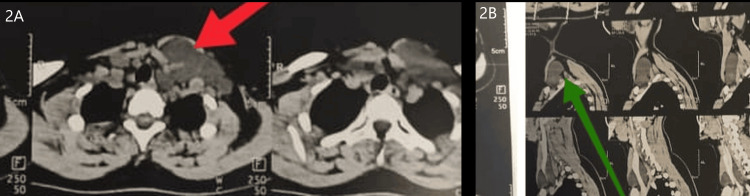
Contrast-enhanced cervicothoracic CT scan of a pediatric patient with cystic lymphangioma. (A) Axial images: the red arrow indicates a large multiloculated hypodense cystic lesion in the left lateral cervical region, well-circumscribed, with mild mass effect on adjacent cervical structures and no solid enhancing components. (B) Sagittal images: the green arrow demonstrates the cervicothoracic extension of the lesion, highlighting its craniocaudal spread across the cervical and superior mediastinal compartments. The overall imaging pattern is consistent with cystic lymphangioma. Digital DICOM images were not available for this case due to limitations in archiving infrastructure at our institution. The CT images presented represent the best available documentation of this case. CT: computed tomography; DICOM: Digital Imaging and Communications in Medicine
